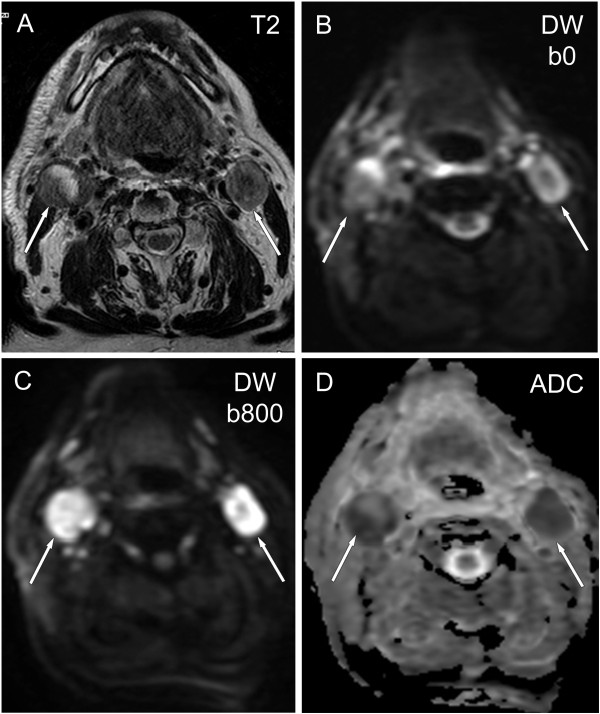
Irradiated oropharyngeal cancer with residual lymphnode enlargement (arrows). A: axial TSE T2-weighted image shows heterogeneous enlargement of the irradiated lymphnodes. B, C, D: Diffusion weighted images in transverse plane demonstrate inhomogeneous restricted diffusion with increased signal on DW-images (B: b value of 50 s/mm^2^, C: b value of 800 s/mm^2^) and decreased signal on ADC map (D) corresponding residual tumour in the nodes.
